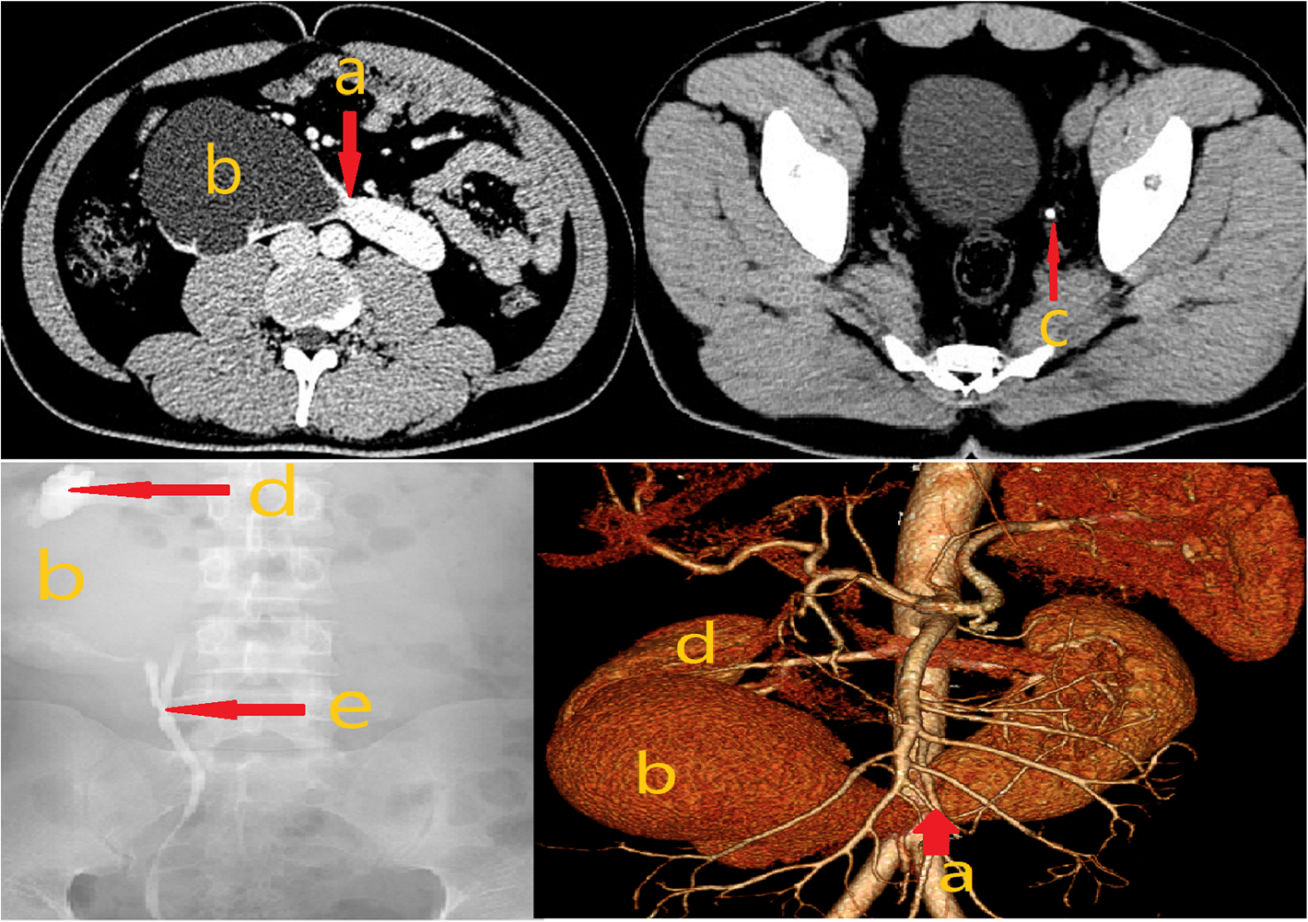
Abdominal enhanced computed tomography (CT), CT ureterography and retrograde urography images show: a) fusion of the bilateral lower poles; b) right lower kidney; c) left ureteral calculus; d) right upper renal collecting system; e) confluence of the duplicate renal collecting system
